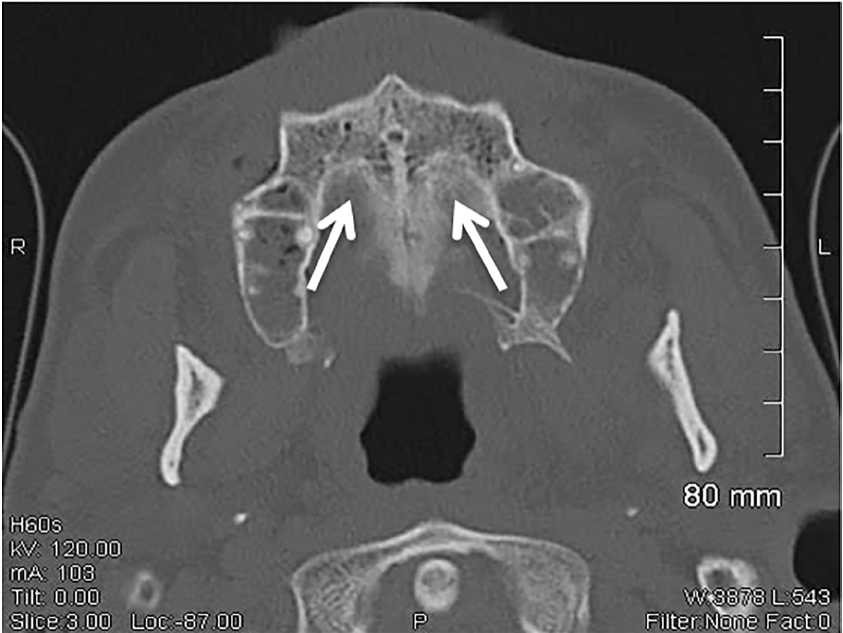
Axial view of a computed tomography scan of facial bones showing cortical irregularity of the hard palate, including submucosal emphysema (white arrows).
